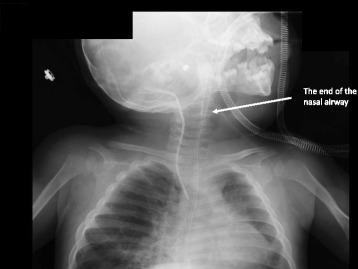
A cervical X-ray at deteriorated respiratory status. A subsequent cervical X-ray revealed that the nasal airway was placed too deeply
